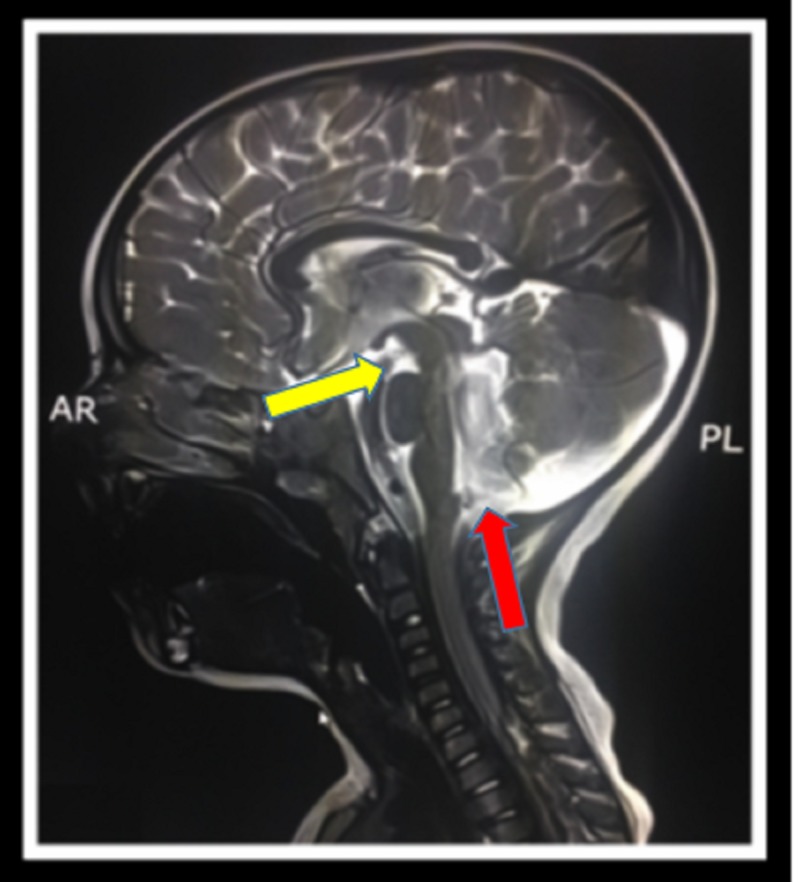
Sagittal T2-weighted MRI AR: Anterior; PL: Posterior; T2: Transverse relaxation time; MRI: Magnetic resonance imaging. Imaging depicts a deep interpeduncular fossa (yellow arrow) and prominent fourth ventricle (red arrow).
